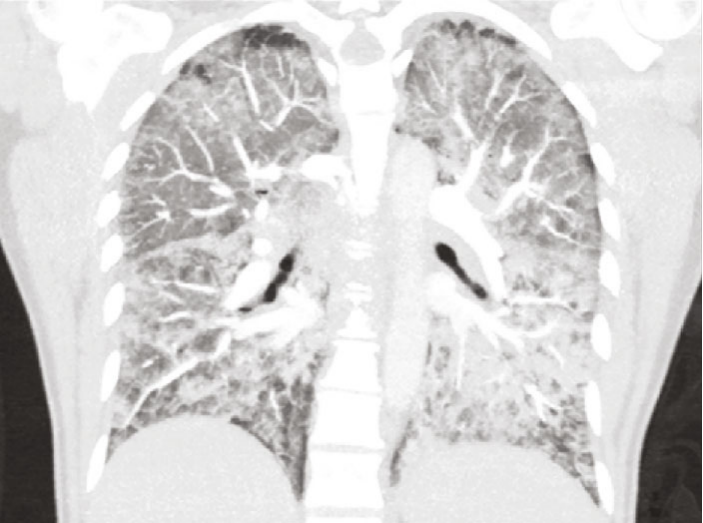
CT angiogram of the chest demonstrating widespread bilateral ground-glass opacities with some basilar and dependent predominance.
